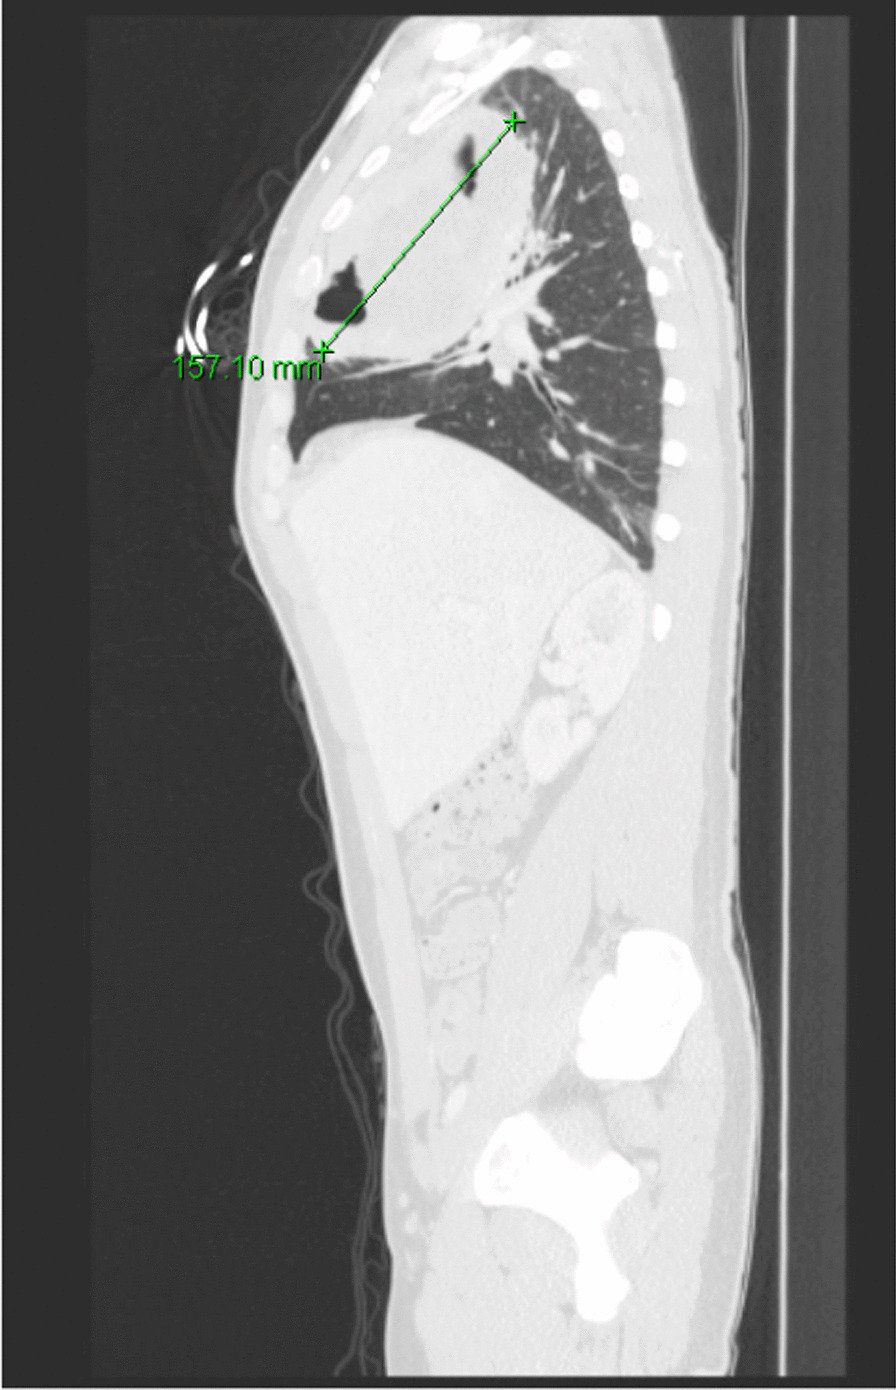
Follow-up CT chest/abdomen/pelvis (sagittal image). Enlarging right upper lobe cavitary lesion
